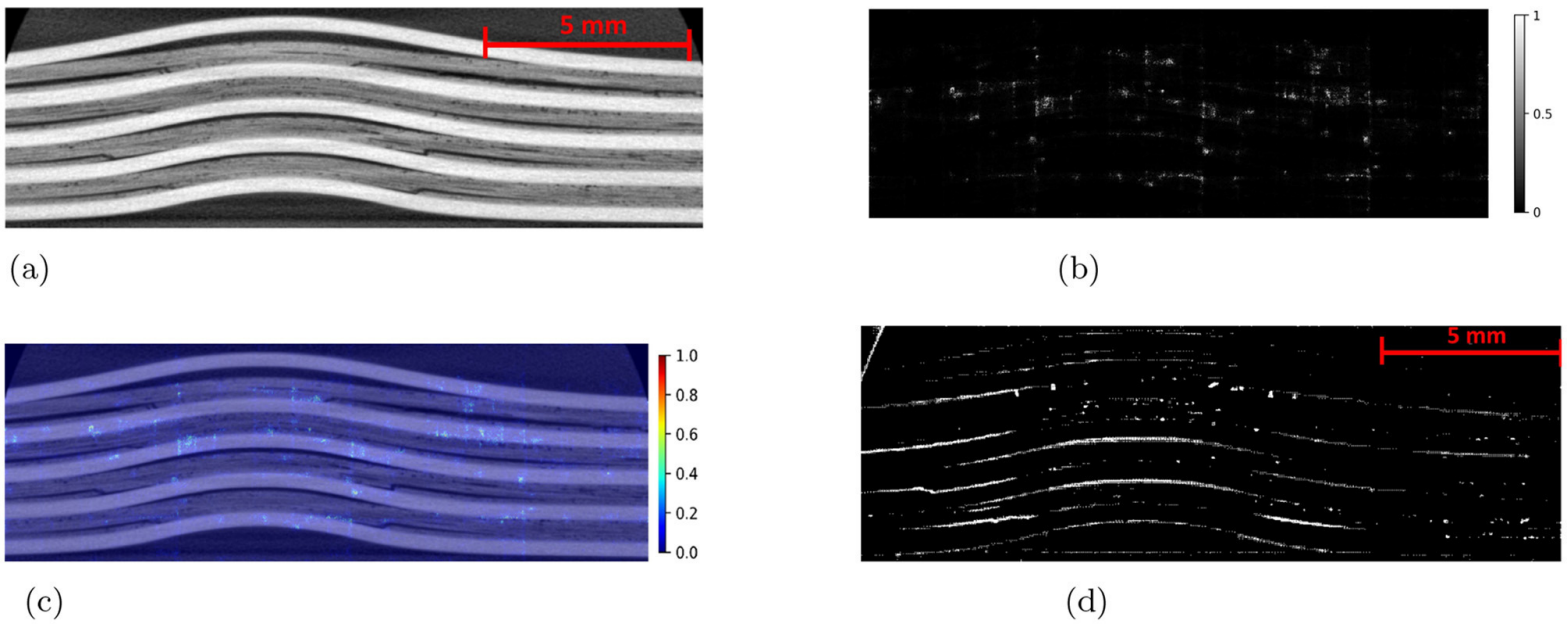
CT slice of the damaged plate with 12.5 Joules impact energy is shown in (a). The attribution map is produced by applying the IG method to the trained SAM. The overlap of the attribution map and CT slice in (c) suggests that the SAM has very low interpretability. (a) CT slice with impact energy of 12.5 Joules. (b) Attribution map. (c) Overlap of attribution map and CT slice. (d) Predicted mask.
